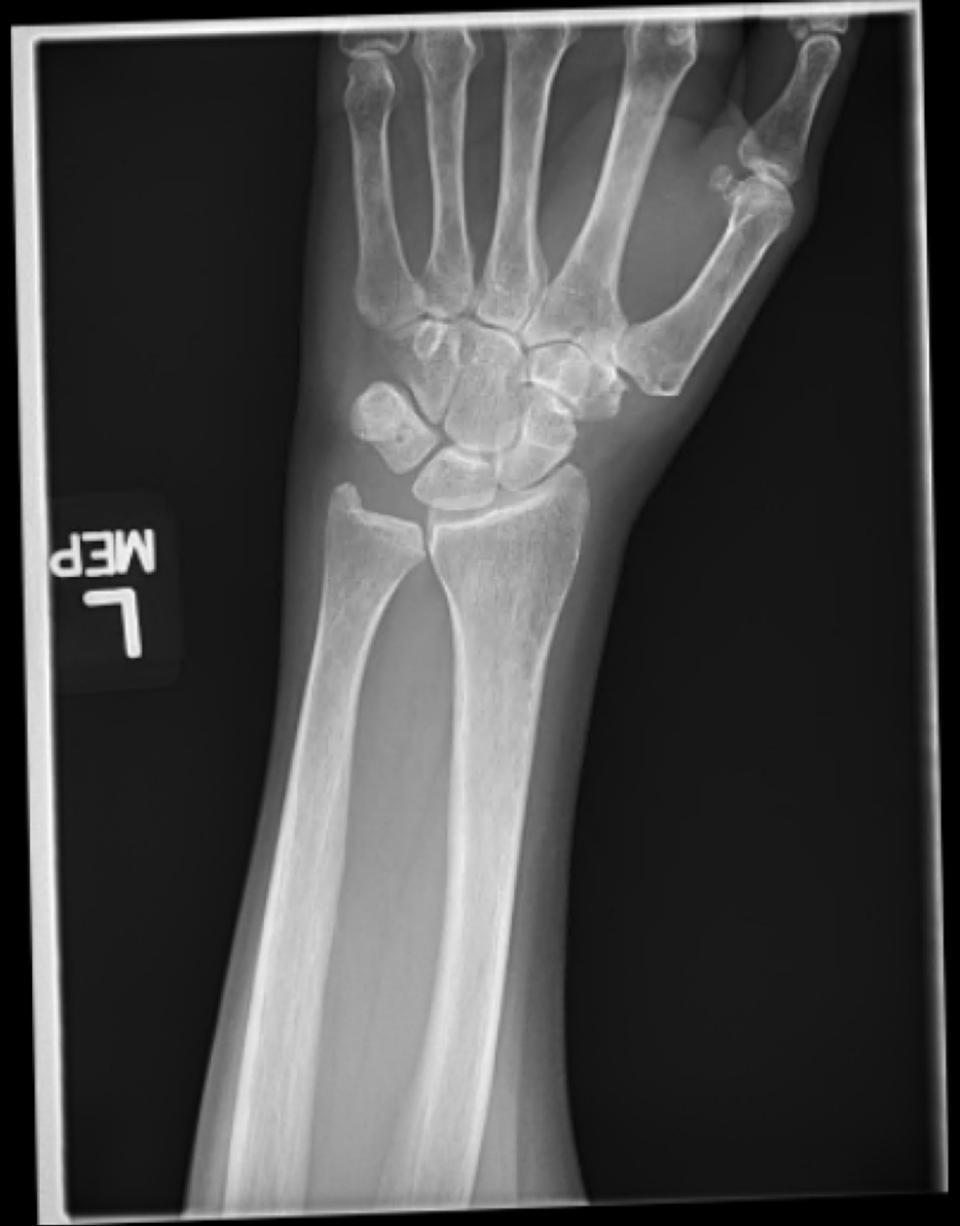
X-ray of patient's left wrist showing some basal joint arthrosis and no evidence of acute trauma.
